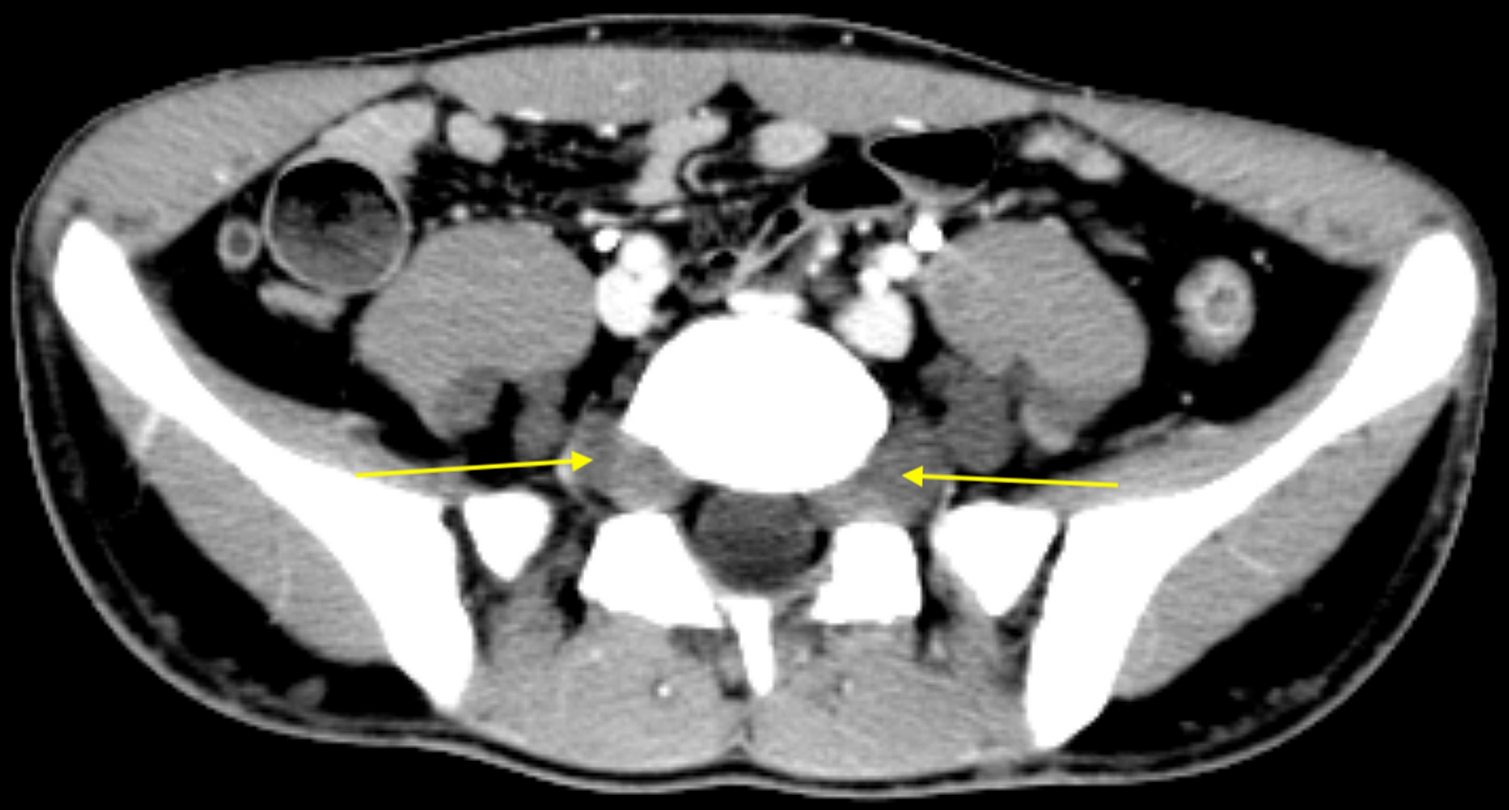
Contrasted axial CT demonstrates bilateral hypoattenuating masses in the parapsoas and presacral regions with homogenous appearance (yellow arrows).
